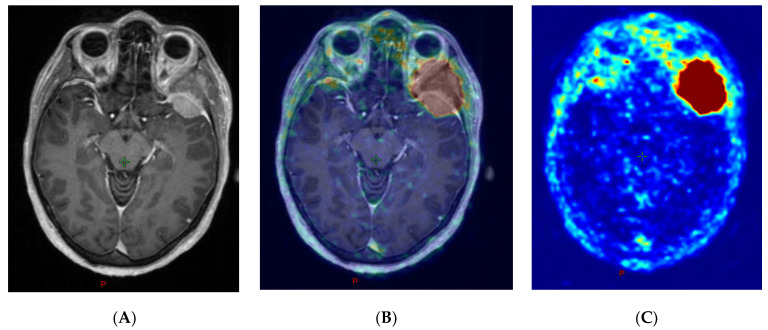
[68Ga]Ga-68-labeled [DOTA0-Phe1-Tyr3] octreotide (Ga-68-DOTATOC)-PET windowing according to MRI. (A) MRI, T1-weighted sequences with magnetization-prepared rapid acquisition with gradient echo; (B) PET windowing orientating on areas with sharp tumor demarcation in MRI using coregistered PET/MRI; (C) attenuation-corrected PET data.
